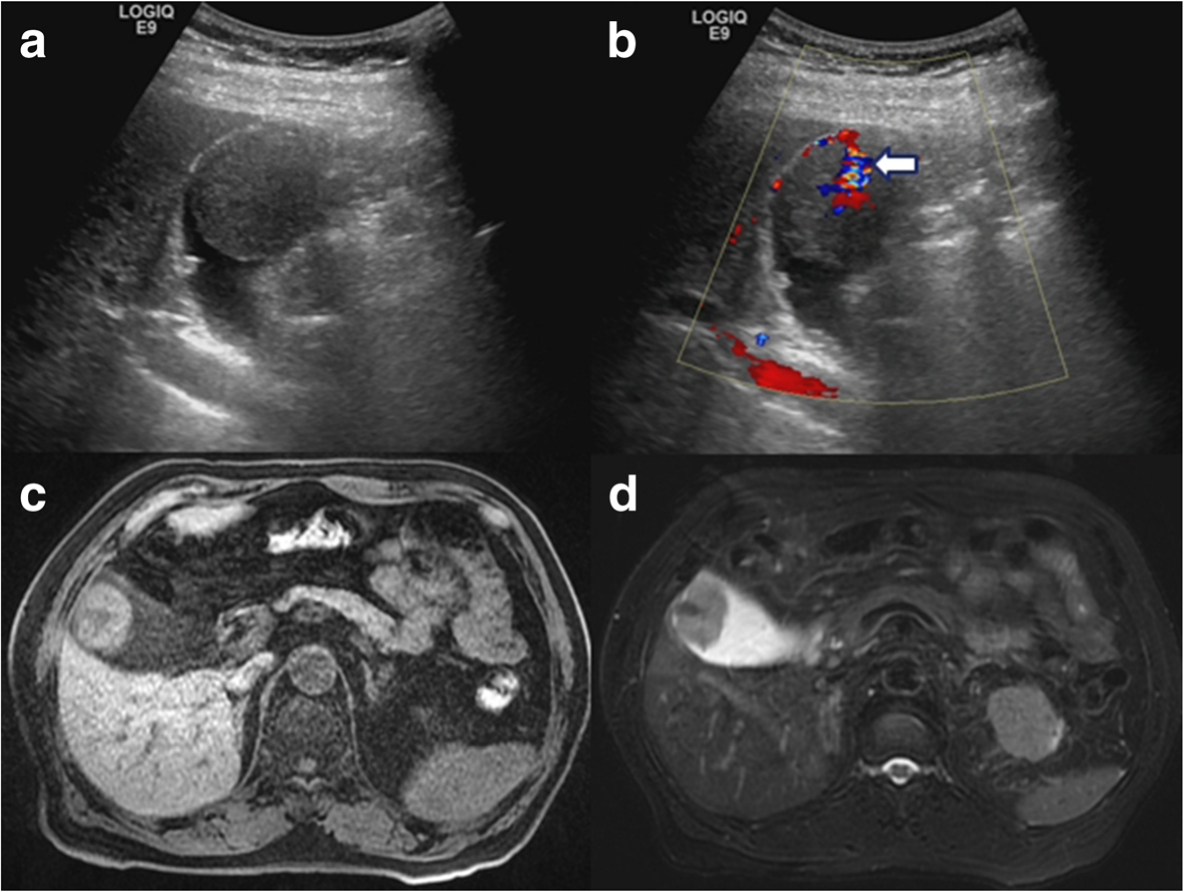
The *gray-scale* (a) and color Doppler sonography (b) show arterial flow (*white arrow*) within the polypoid mass from the cystic artery. On the T1-weighted fat-saturated spoiled gradient recalled echo (c) and fat-saturated T2 fast spin-echo MRI images (d), the polypoid gallbladder tumor demonstrates intermediate signal intensity on T1-weighted image and slightly high on T2-weighted image to that of liver parenchyma
